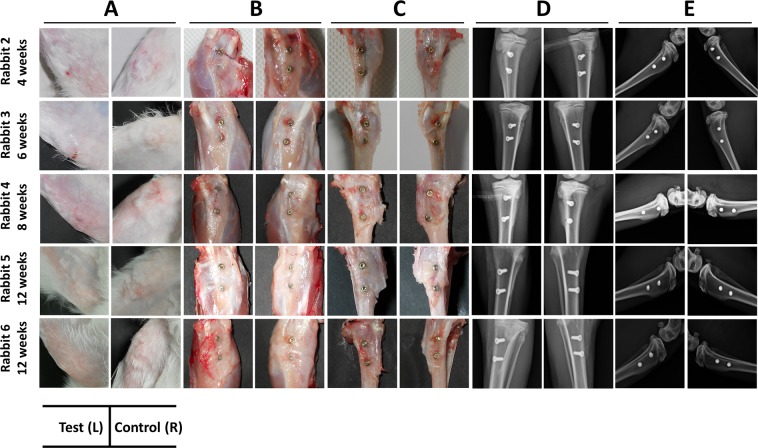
Macroscopic and radiographic images of healed tibia at the end of respective time points. (A) Gross/macroscopic images of skin around implant and peri-implant region, (B) subcutaneous region after excision of skin, (C) exposed implants after excision of muscles and soft tissues, (D) anterior-posterior radiograph of tibiae, (E) medio-lateral radiograph of tibiae. The test implants have not exerted any untoward influence on peri-implant area, as suggested by absence of discolouration, osteolysis and rejection reaction etc. Hence, no complications in healing or occult defects with respect to these implants were noted.
